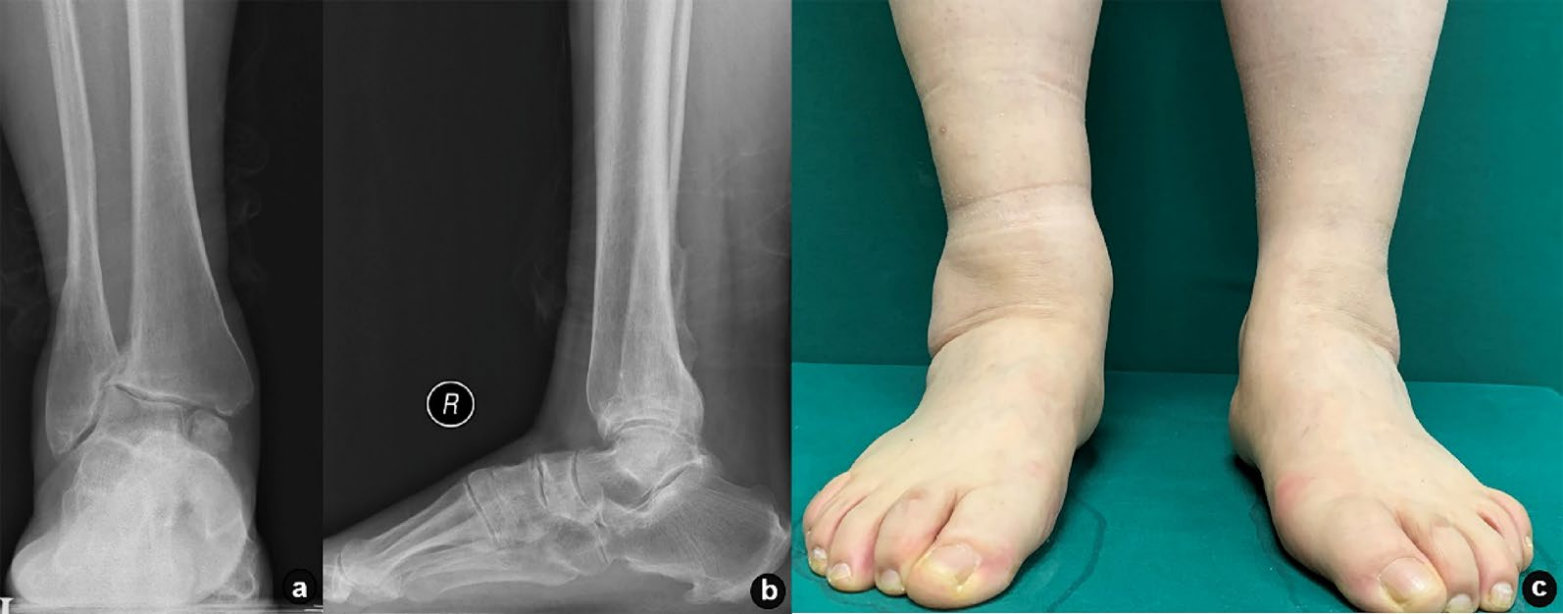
Preoperative radiographs of a specific case. Female, 55 years old, 13 years of conservative treatment for ankle fracture with valgus ankle arthritis. a, b Preoperative weight-bearing anteroposterior and lateral X-rays of ankle joint; c preoperative positioning images showing ankle valgus
